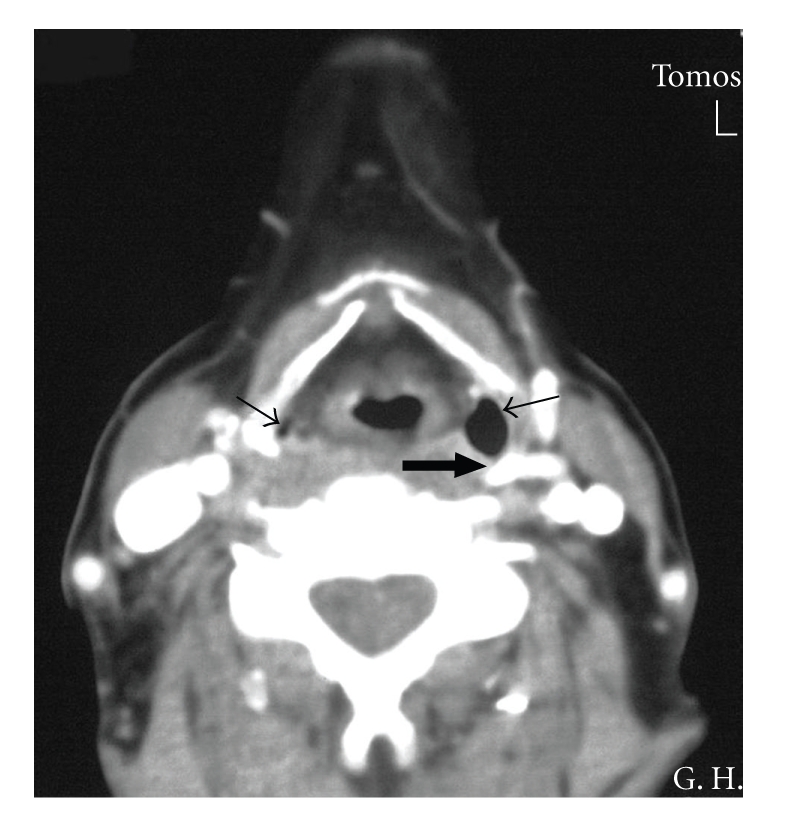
CT neck. Axial cut with contrast at the level of C5 vertebra. Rotation of thyroid cartilage, black arrow shows the left thyroid cornu. The airfilled cavity next to the left thyroid cornu is the left piriform sinus which is clearly bigger than the right one due to the rotation of the larynx (black thin arrows).
